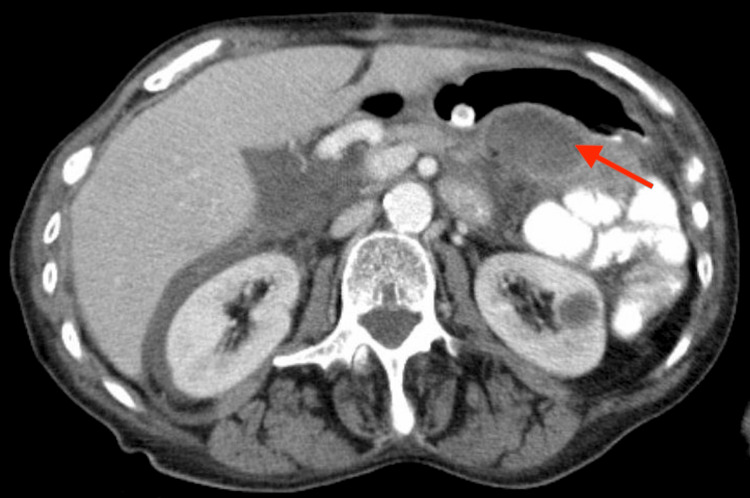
Axial computed tomography view of the abdomen and pelvis with contrast, showing the postoperative peritoneal abscess (red arrow)
